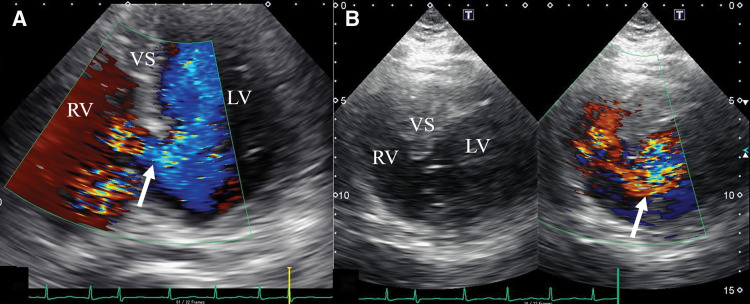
Transthoracic echocardiographic findings. Apical (A) and short-axis (B) views show a left-to-right shunt jet (arrows) from the left ventricle to the right ventricle. LV, left ventricle; RV, right ventricle; VS, ventricular septum
